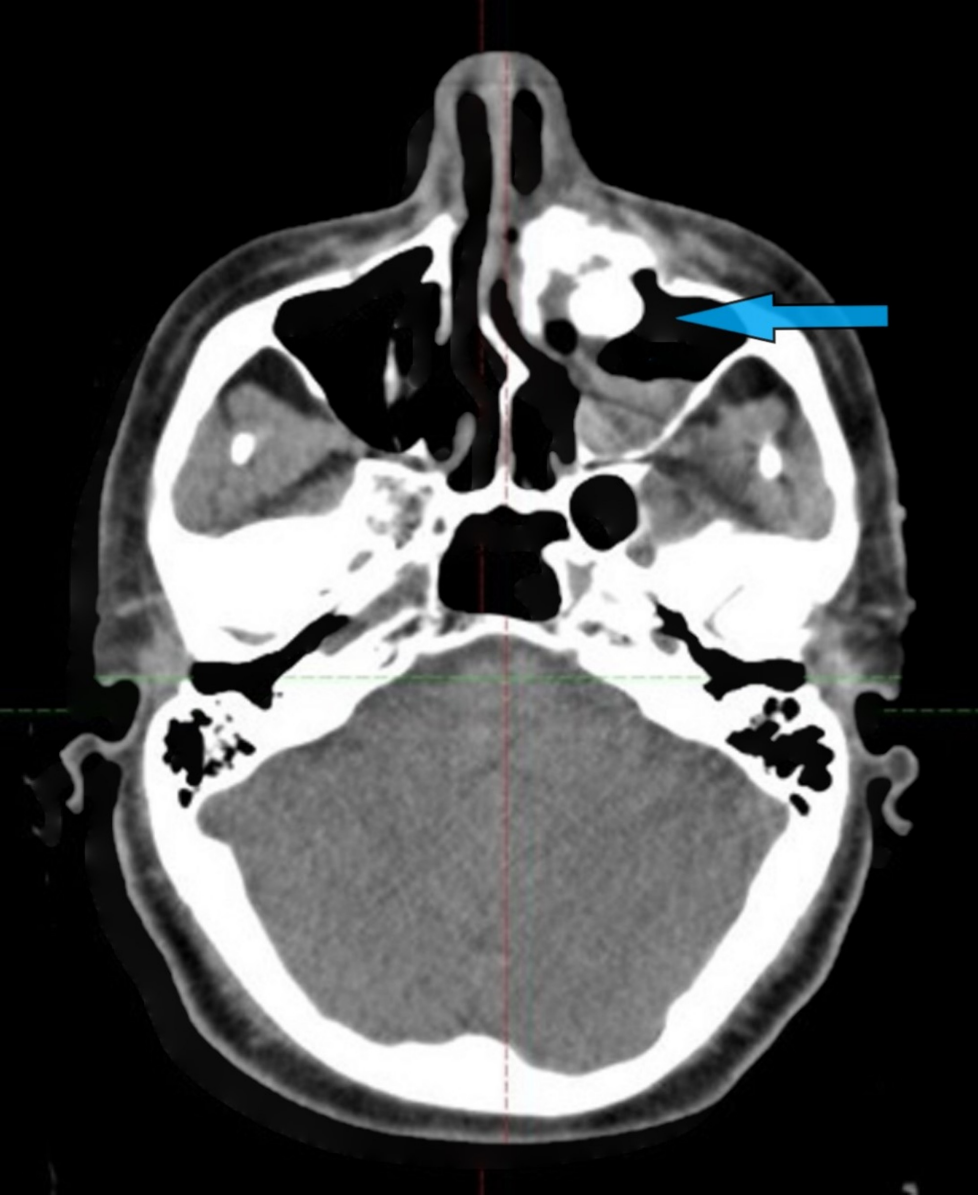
CT evaluation of response at the 5th week of concurrent CCRT of left PSNNECs shown by arrowhead as the optimal radiological resolution CT: computed tomography; CCRT: chemoradiotherapy; PSNNEC: primary sino-nasal neuroendocrine carcinoma
